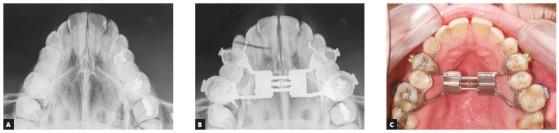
Initial occlusal radiograph (A). Occlusal radiograph (B) and intraoral photograph (C) after surgically-assisted rapid maxillary expansion.
